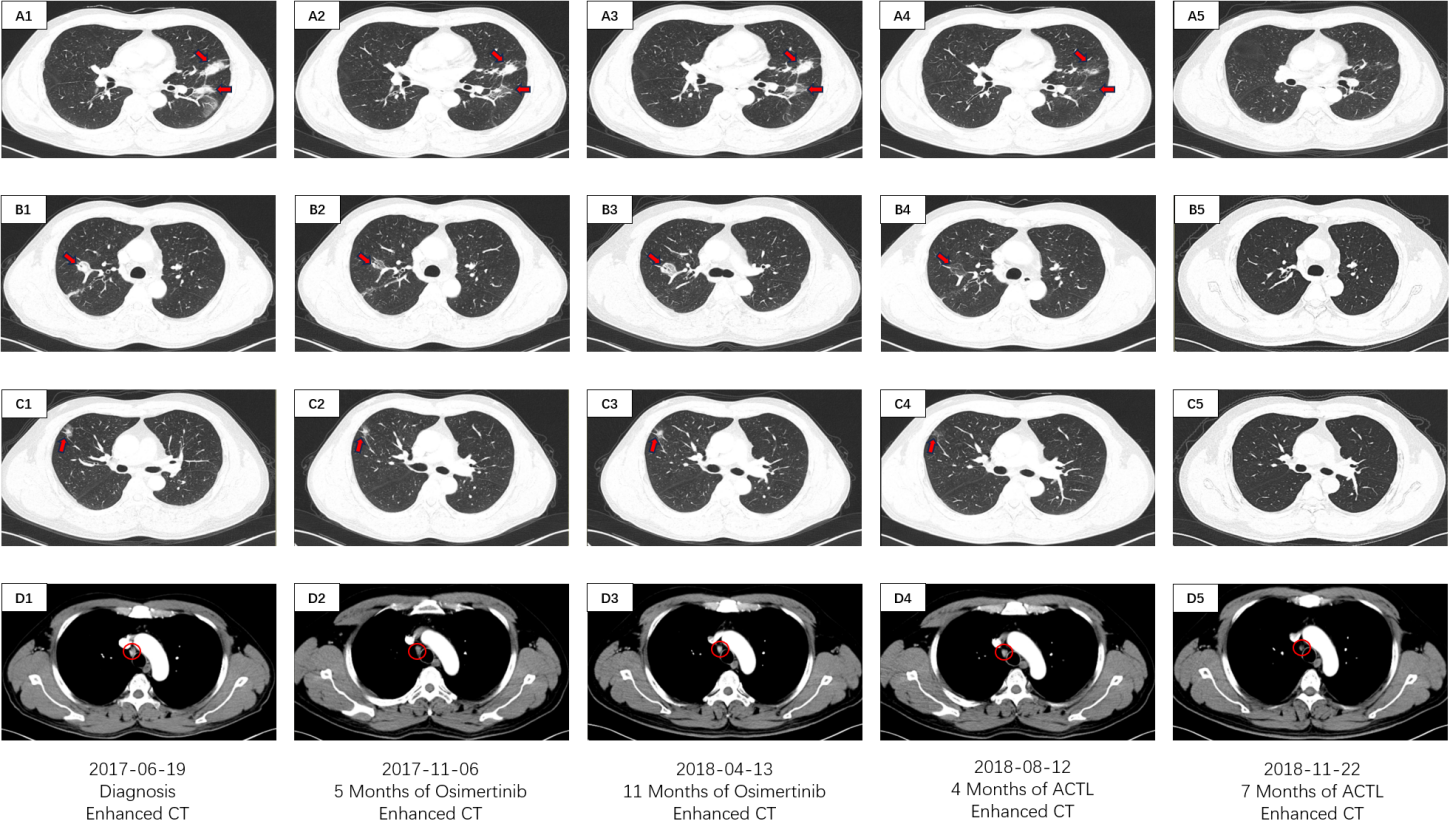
CT imaging assessment of lung lesions and mediastinal lymph node throughout the course of disease corresponding to their respective time points. (A1–D1) CT imaging of the lung window (A1–C1) and the mediastinal window (D1) on June 19, 2017; (A2–D2) CT imaging of the lung window (A2, B2, C2) and the mediastinal window (D2) on November 06, 2017; (A3–D3) CT imaging of the lung window (A3–C3) and the mediastinal window (D3) on April 13, 2018; (A4–D4) CT imaging of the lung window (A4–C4) and the mediastinal window (D4) on August 12, 2018; (A5–D5) CT imaging of the lung window (A5–C5) and the mediastinal window (D5) on November 22, 2018. (A1–5) images manifests the lesions of the left lobe in the CT lung window. (B1–5) images manifests the inner lesion of the right middle lobe in the CT lung window. (C1–5) images manifests the outer lesion of the right middle lobe in the CT lung window. (D1–5) images manifests the mediastinal lymph nodes in the CT mediastinal window.
